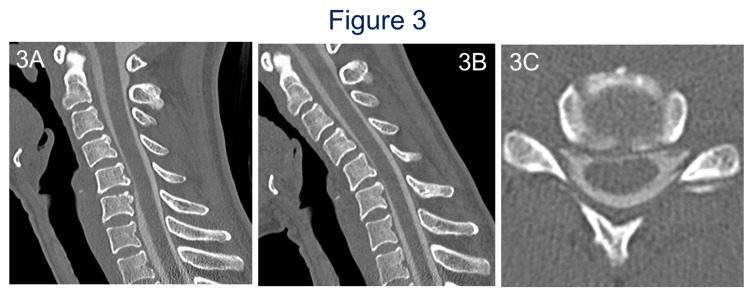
Preoperative CT myelography of case 2. CT myelography showed neutral position sagittal view (A) and anteflexion sagittal view (B), axial view at C5-6 in a flexed position (C) revealing anterior shift of the spinal cord and the spinal cord contacting vertebrae at the C5-6 level with the cervical spine in only flexed position. CT, computed tomography.
